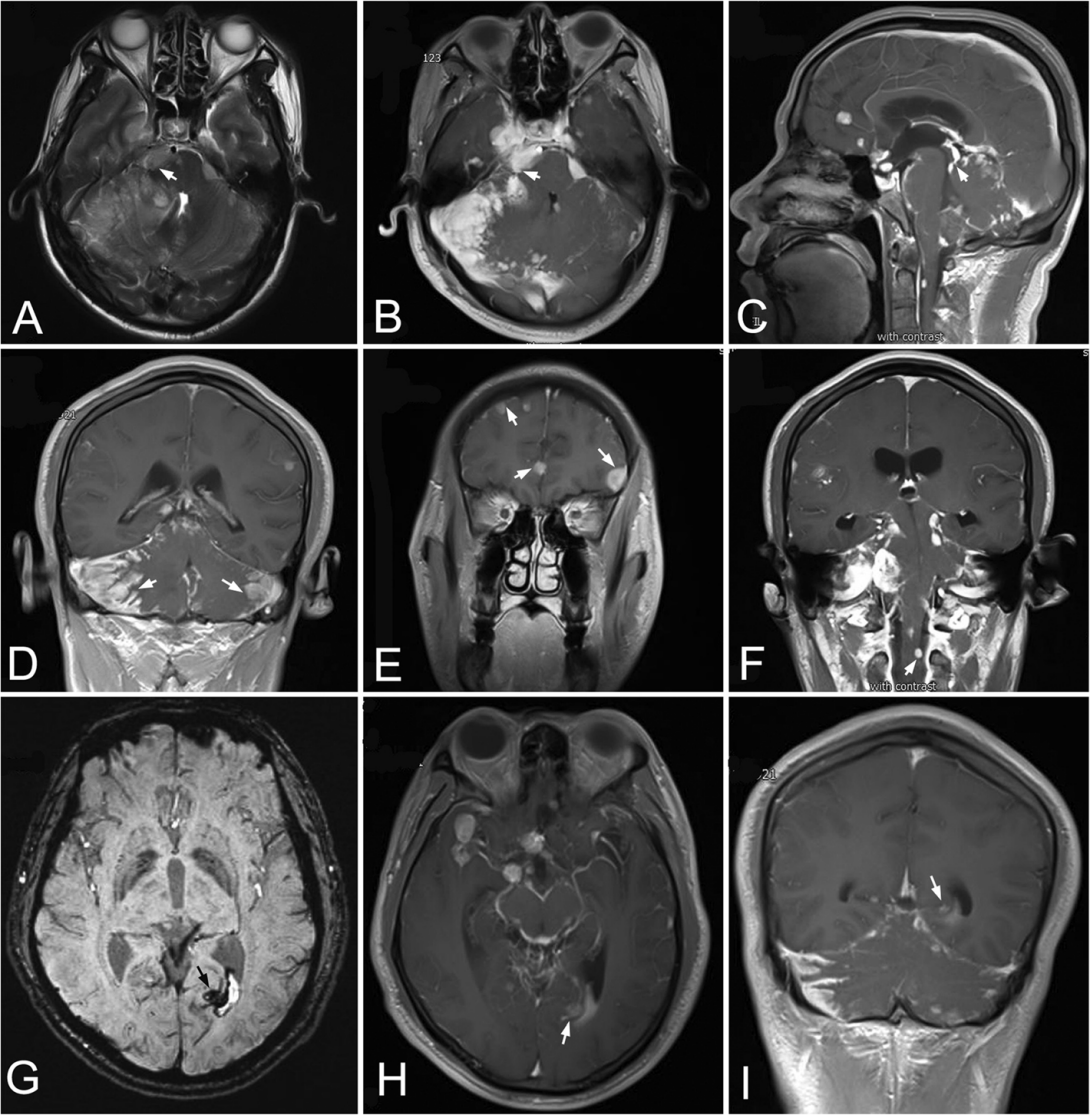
The features of cerebral MRI. MRI showed multiple abnormal patchy signals with marked enhancement in bilateral cerebellopontine angle cistern (a, b, arrow), quadrigeminal cistern (c, arrow), bilateral cerebellum (d, arrow), cerebral hemisphere (e, arrow), and upper cervical cord surface (f, arrow). SWI showed the hemorrhagic lesion located at the in-depth of left calcarine fissure (g, arrow), which was confirmed by the axial (h, arrow) and coronal (i, arrow) enhanced MR imaging
